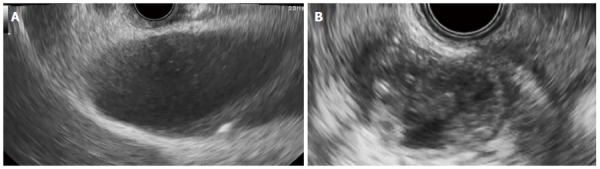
Endosonographic image. A: Endosonographic image before gallbladder drainage. Contents in the gallbladder were mainly sludge without apparent gallstones; B: Endosonographic image at the time of recurrence of cholecystitis. Sludge volume in the gallbladder was more increased than when gallbladder drainage was performed.
